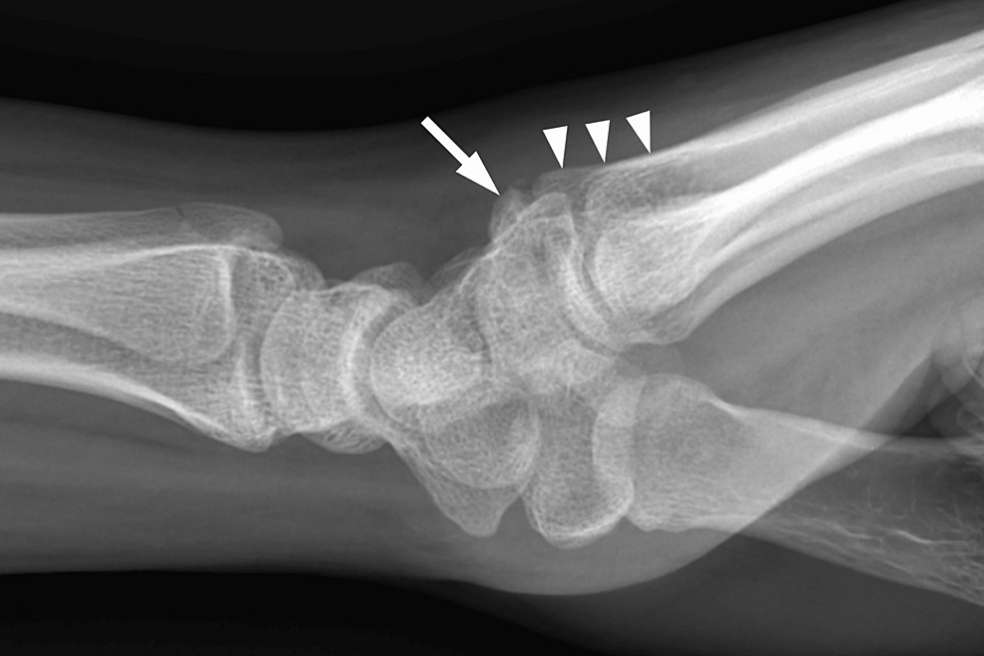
Lateral radiographic projection of the symptomatic wrist demonstrating a small ossicle (arrow) adjacent to the base of the third metacarpal (arrowheads).
